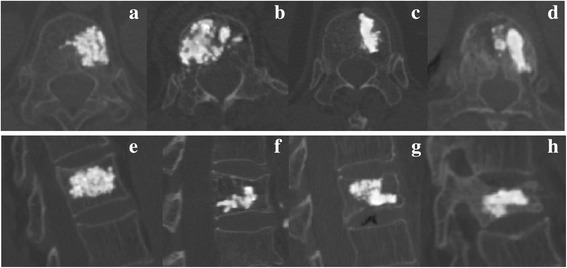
Computed tomography images of 4 patients with T12 osteoporotic vertebral compression fractures after vertebral augmentation. a–d Cross-section images of grade 0–3 vertebral height; e–h sagittal images of grade 0–3 vertebral height
